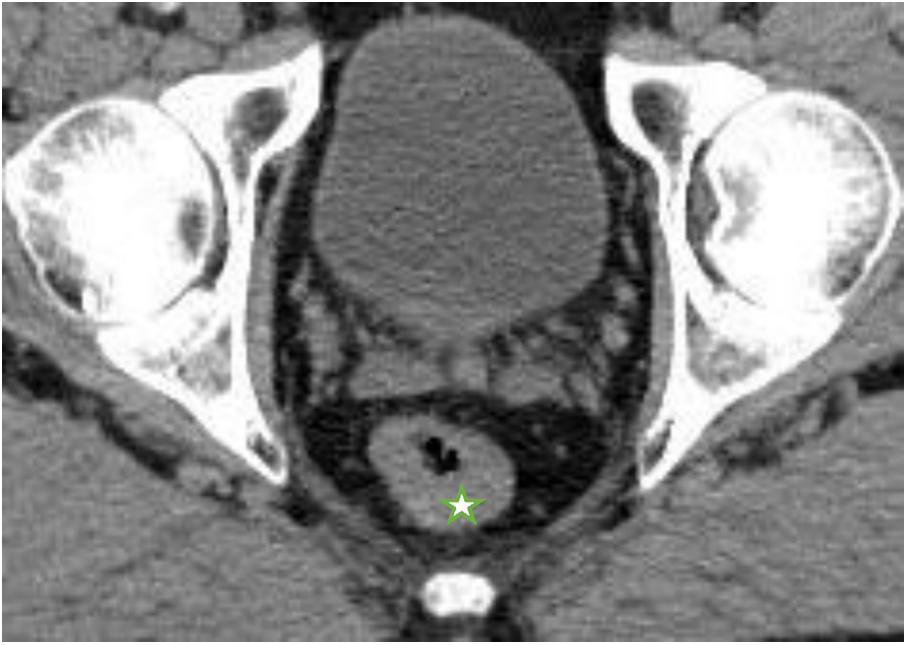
Computed tomography (February 2017) showing wall thickening of the rectum, marked with a star.
